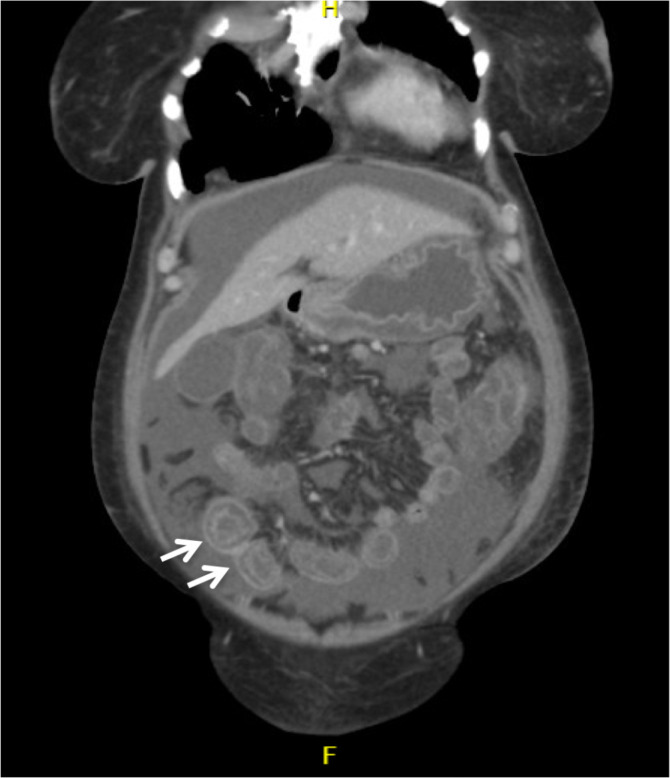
Axial CT Angiogram of the abdomen shows bilateral pleural effusion, right side more than left side (arrow).
